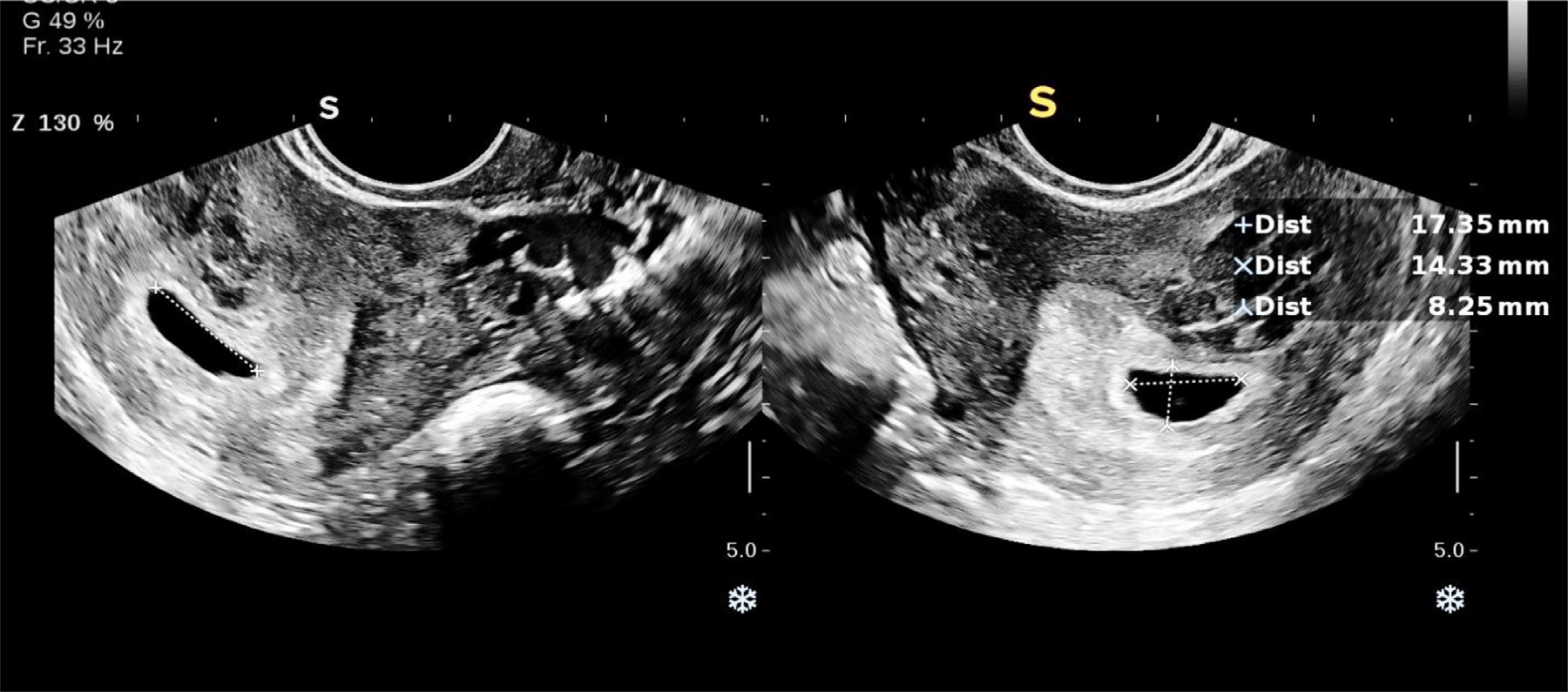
Transvaginal ultrasound revealed a gestational sac in the proximal uterine cavity containing a yolk sac but no fetal pole (FP).
